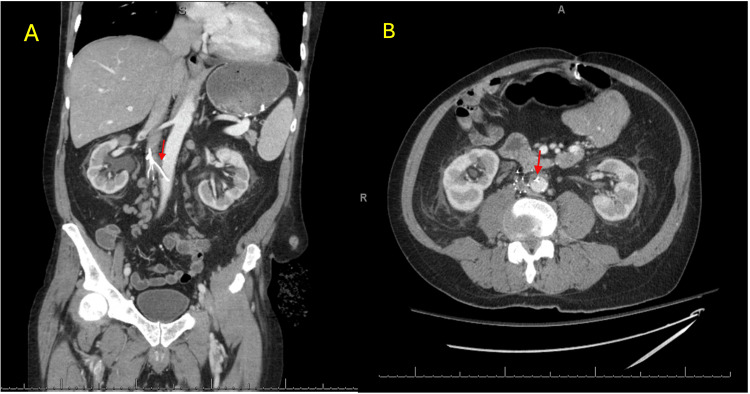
Computed tomography (CT) scan demonstrating IVC filter penetration into the aortic lumen A) Coronal view with the red arrows demonstrating the site of aortic penetration with no extravasation. B) Axial view with the red arrows demonstrating the site of aortic penetration with no extravasation.
